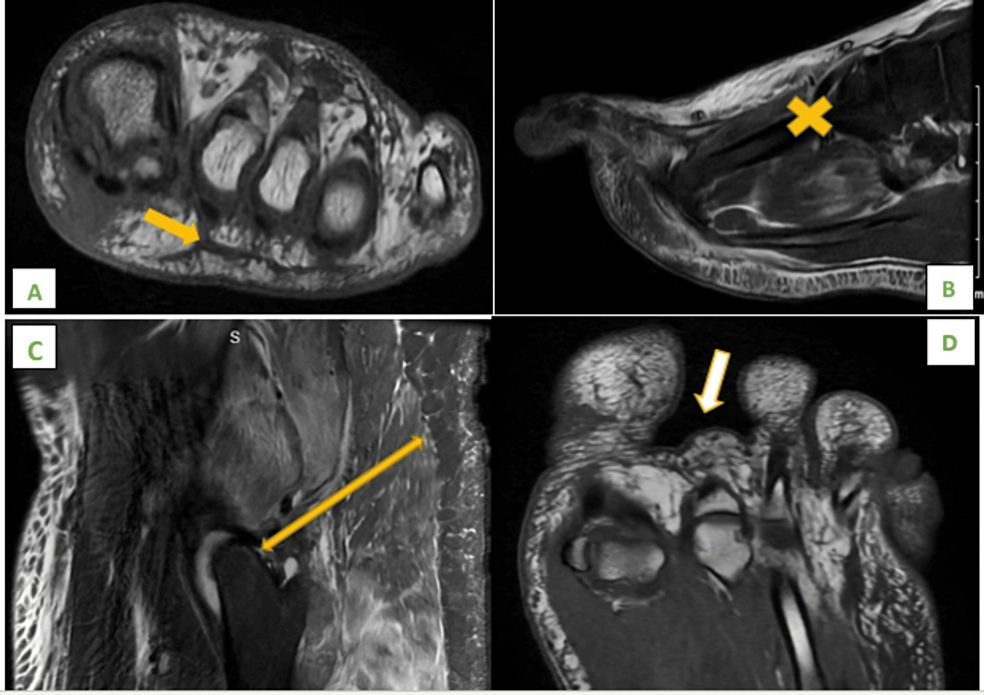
MRI of the left foot and left hip. A: Joint effusions are seen throughout the interphalangeal joints and metatarsophalangeal joints. B: Minimal first and second intermetatarsal space bursitis + small amount of fluid surrounding the plantar fascia. A large amount of soft tissue edema on the dorsal aspect of the left foot. C: Enlargement as well as edema of the left gluteus minimus, gluteus medius, gluteus maximus, quadratus femoris, abductor magnus, and piriformis muscles with edema coursing in the superficial as well as deep fascial planes of the muscles surrounding the left hip extending into the left hemipelvis due to myositis. D: Edema throughout the intrinsic muscles of the foot as well as throughout the deep and superficial fascial muscular planes.
